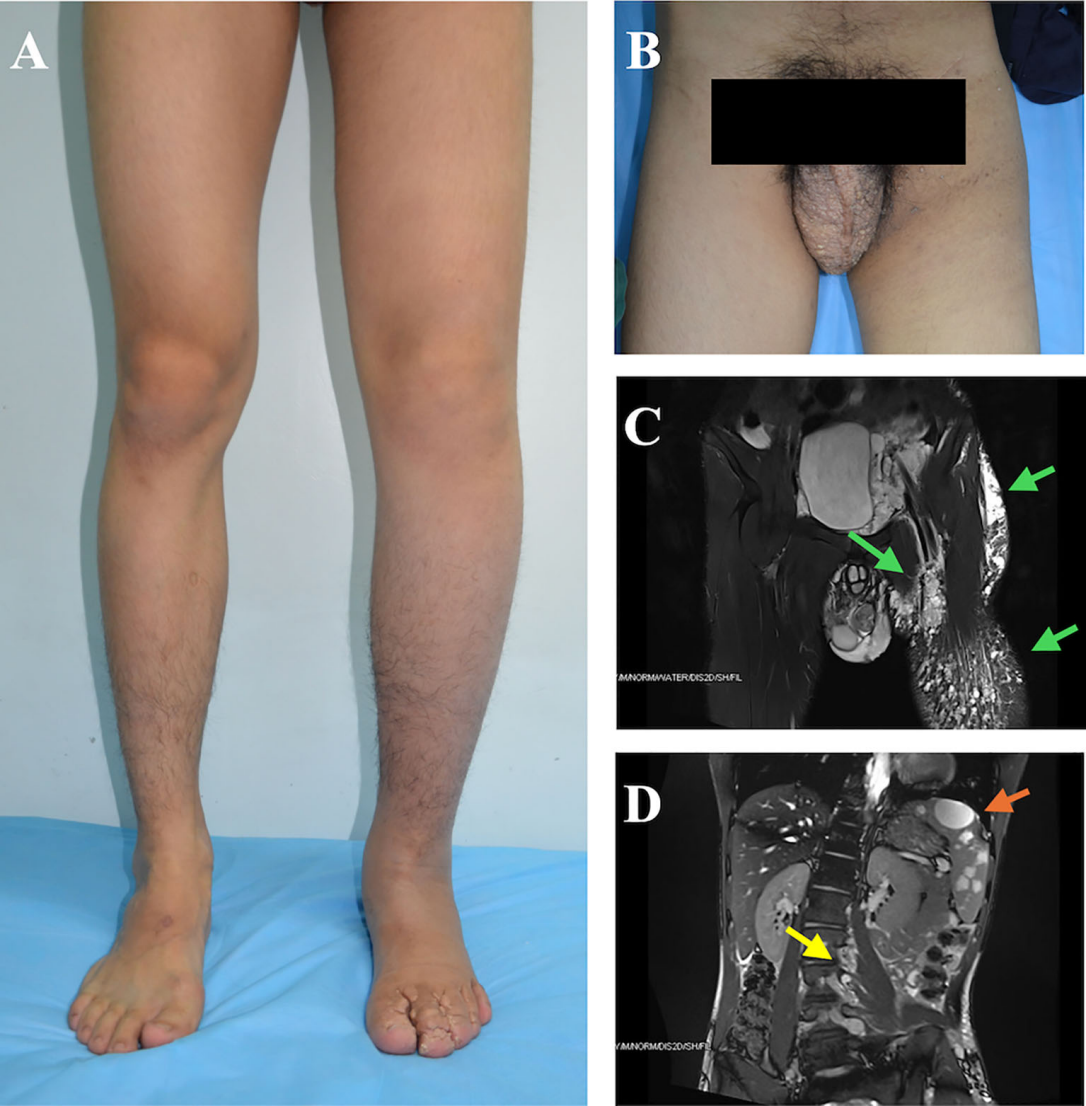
A male patient with generalized lymphatic anomaly (GLA) manifested cystic lesions of the spleen and lymphedema involving the left lower extremity and perineal region. (A, B) Clinical photographs demonstrate extensive swelling of the left lower extremity and perineal region, with prominent lymphorrhagia involving the penis, scrotum, and interdigital spaces of the left foot. Localized skin thickening and textural changes are evident. (C) Coronal T2-weighted MRI reveals multicystic and nodular high-signal-intensity lesions in the left perineal region and left lower extremity, involving the dermis, subcutaneous tissue, and deep fascia. Perilesional soft tissue edema is noted (green arrows). (D) Coronal T2-weighted MRI demonstrates multiple well-circumscribed, round hyperintense lesions within the spleen (orange arrows). Additionally, multiple tortuous dilated vascular structures are observed in the thoracolumbar spinal canal (yellow arrows).
